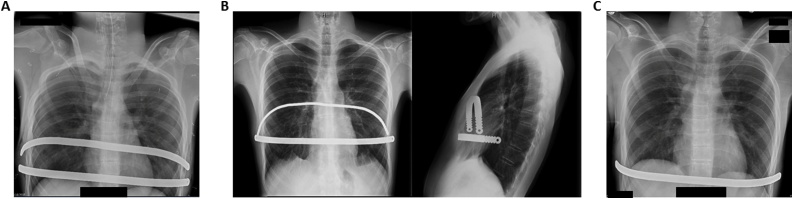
A, Postoperative anteroposterior chest radiograph showing two pectus bars in place. B, Posteroranterior and lateral chest radiographs showing bar slippage. C, Anteroposterior chest radiograph after removal of slipped bar.
